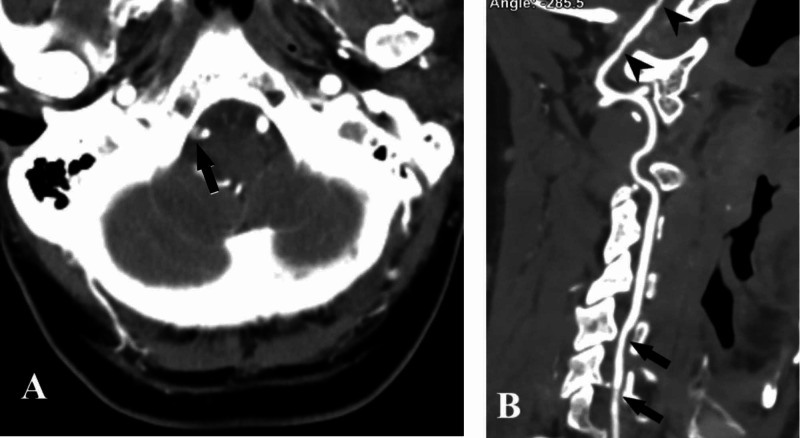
CTA findings – case 2 CTA of the right vertebral artery showing a double-lumen sign in (V4) segment (arrow) in the axial view, while sagittal reconstructed view (B) shows multiple stenotic lesions in V1 (head arrows) and in V1-V2 (arrows) CTA: computed tomography angiography
